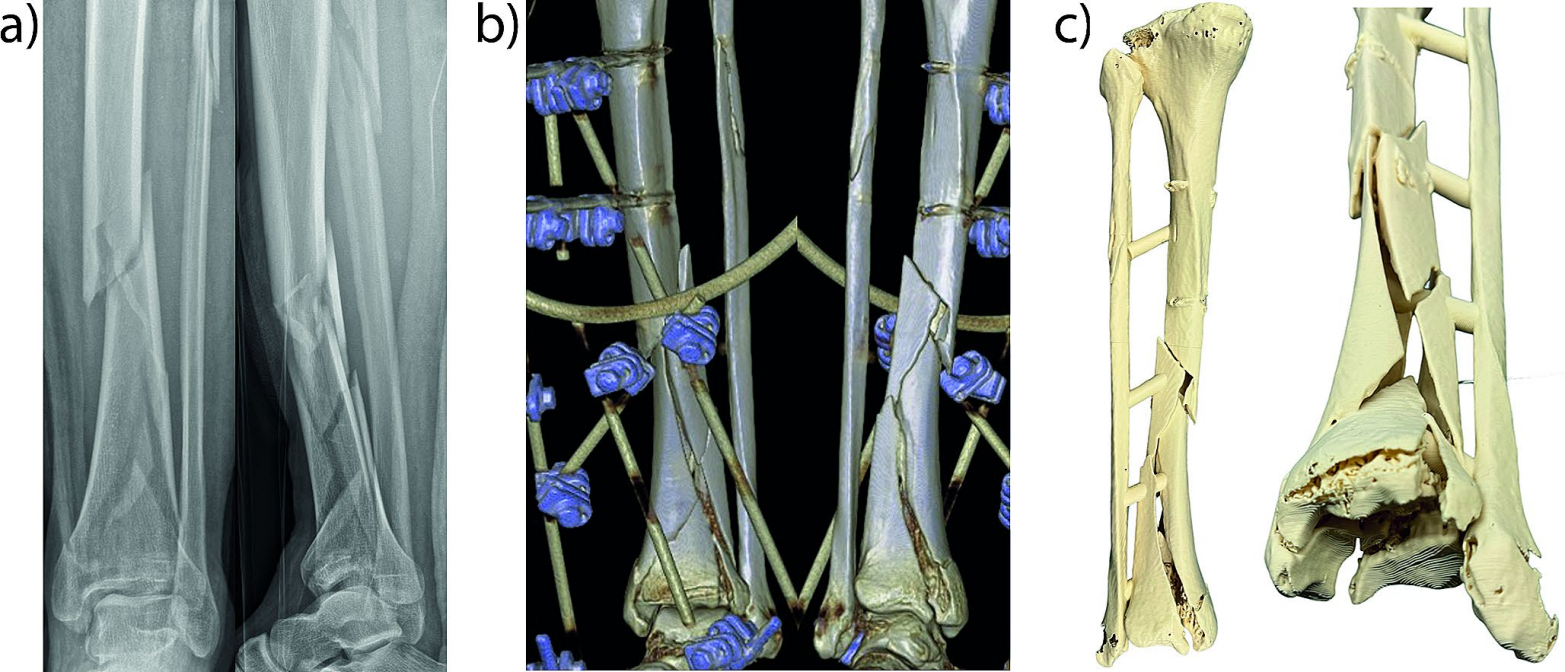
Radiograph, 3D reconstruction, and 3D print of an open fracture of the tibia (Case 1)
